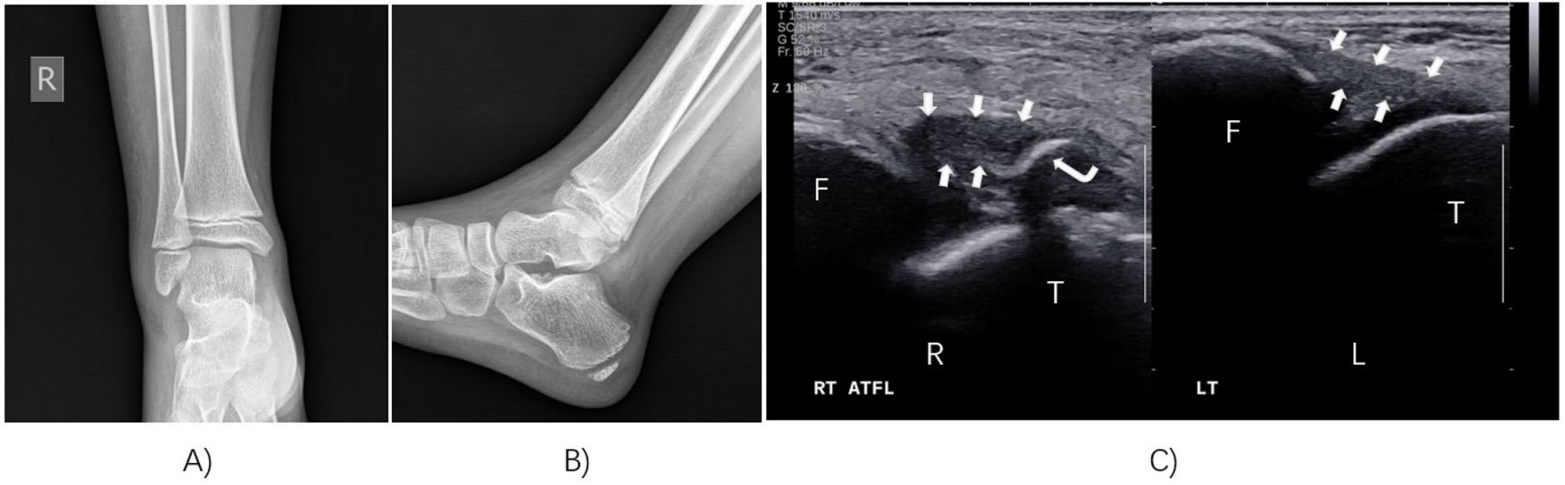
Chondral avulsion fracture of the lateral talus process was negative on radiography but positive on ultrasonography. (A) Anteroposterior X-ray radiographs of the ankle. (B) Lateral X-ray radiographs of the ankle. (C) Ultrasound examination of the ankle was performed and compared with the contralateral side. *R*, right; *L*, left; *F*, fibula; *T*, talus; arrowhead, anterior talofibular ligament; right angle arrow, chondral avulsion fracture of the lateral talus process
